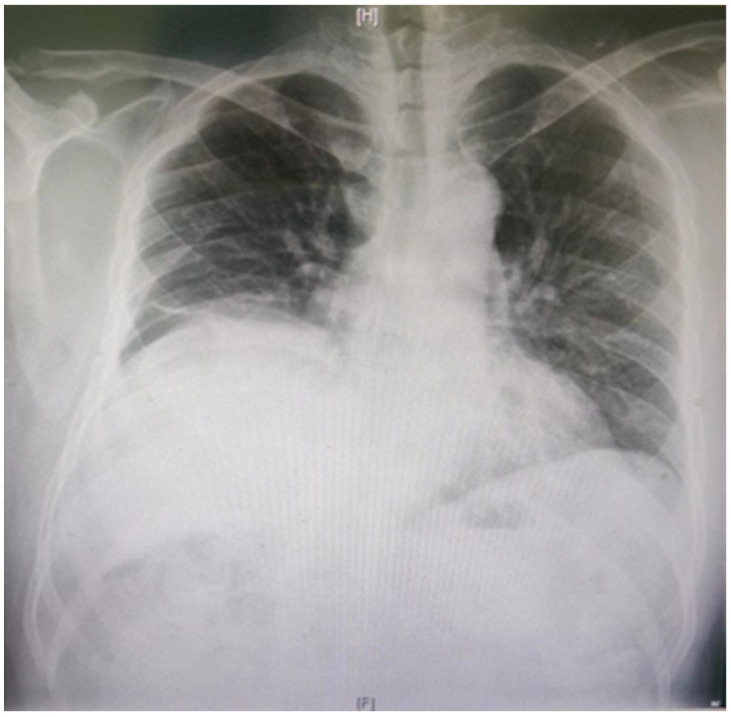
The chest radiograph shows the pathological elevation of the right hemidiaphragm.
